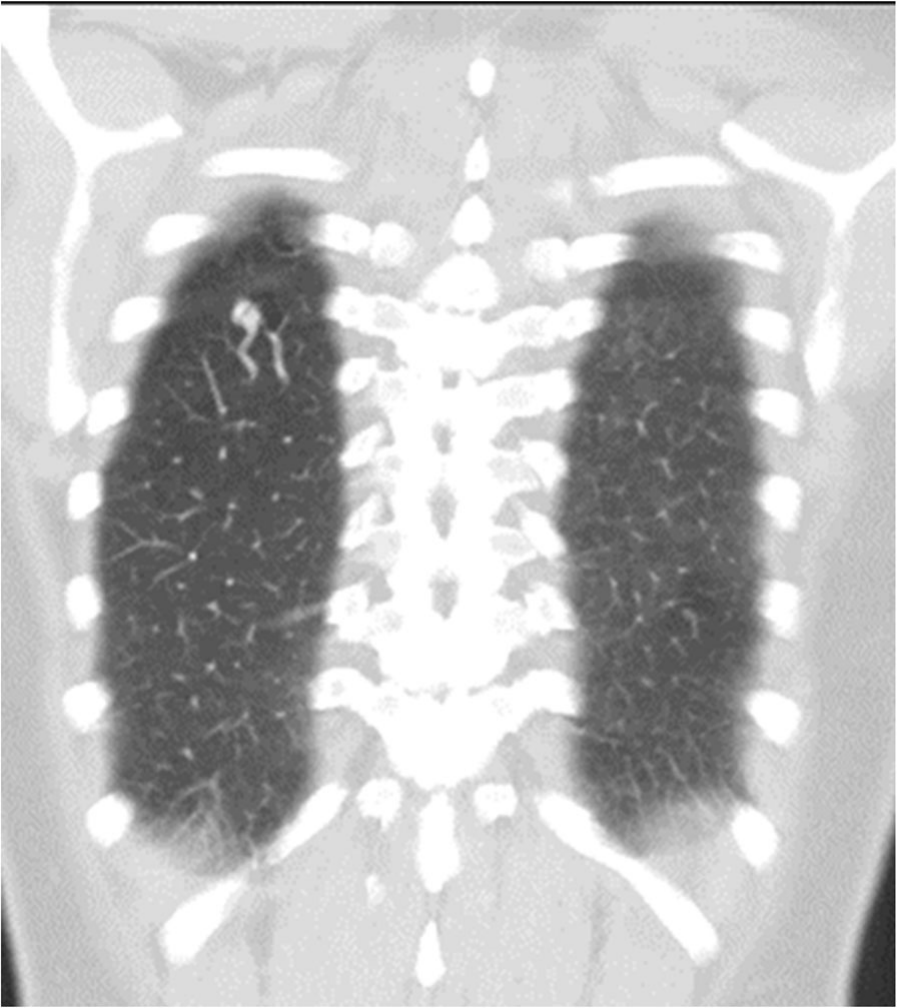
Non-contrast CT showed a simple PAVM in right upper lobe with feeding artery of 3, 3 mm in diameter
